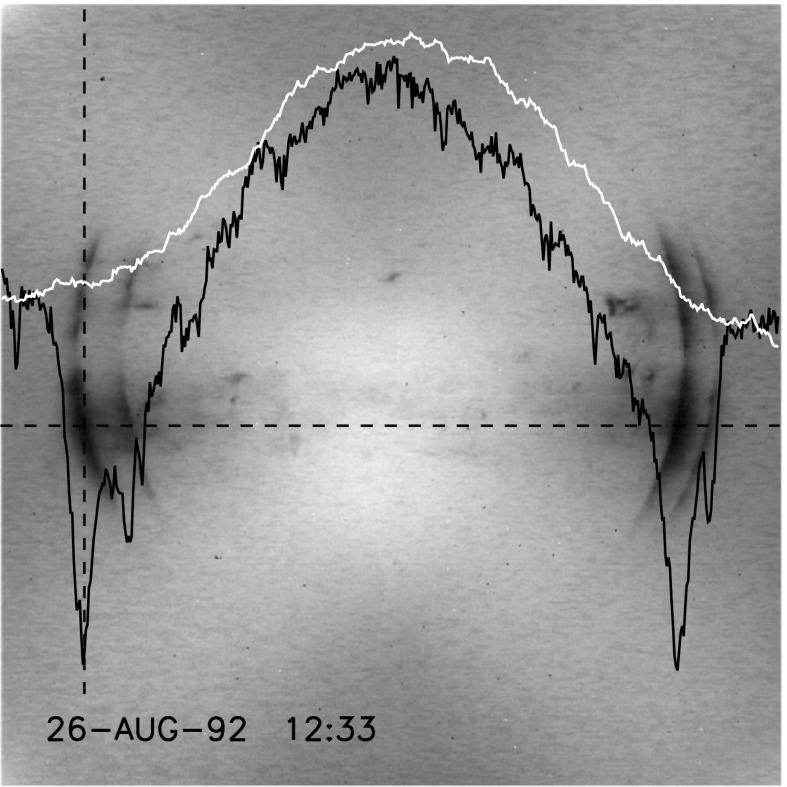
Demonstration of decrease in CCD optical sensitivity caused by soft X-ray damage after nearly one year of use. Image scaled to reveal details. Curves are described in text.
